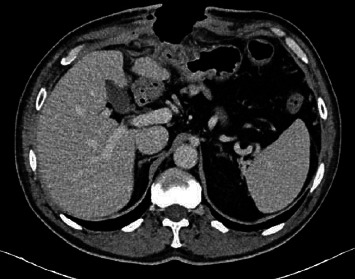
Axial CT portal venous phase section shows a large discontinuity at the level of the anterior abdominal wall, as a result of surgical drainage of the previously noted collection. The postoperative CT revealed a good surgical cleaning of the site, with no further collections observed and no postoperative complications identified.
